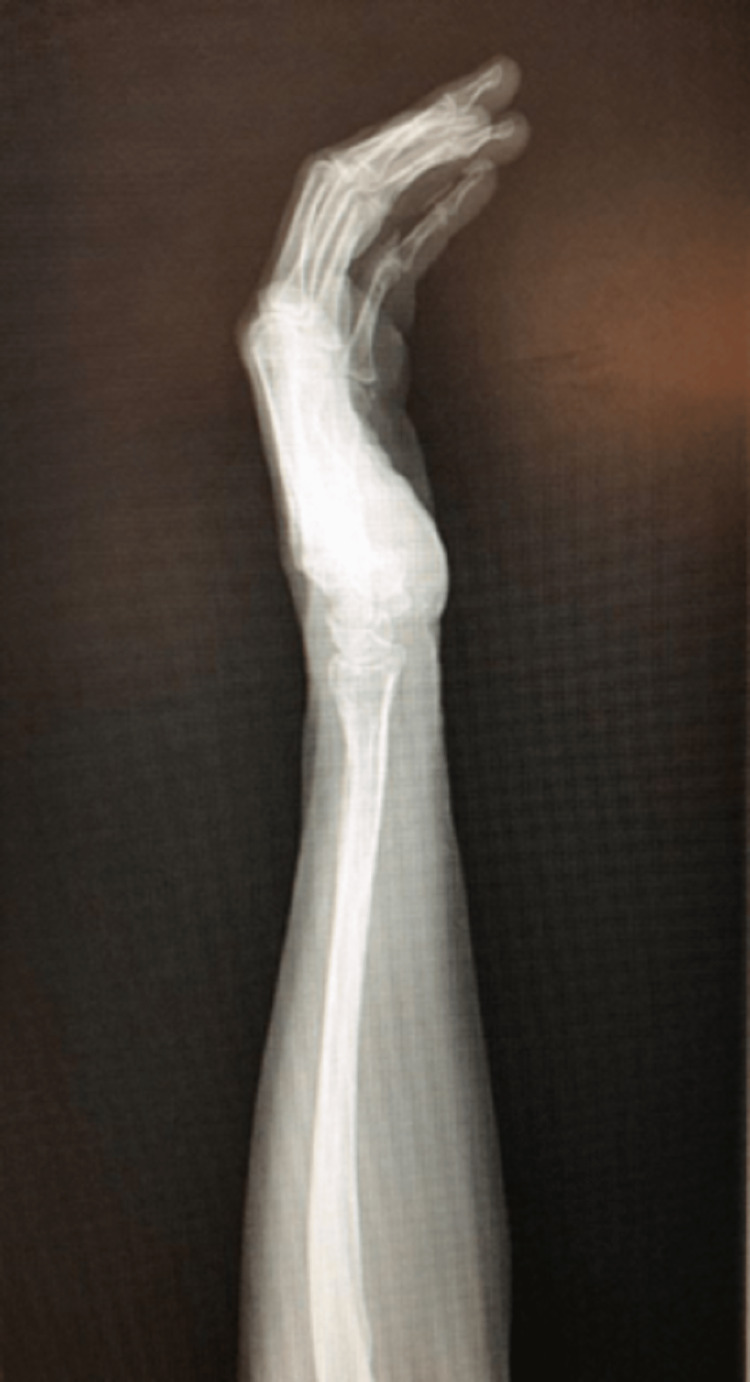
Left forearm and wrist radiograph - lateral view Radiograph of the patient’s left distal forearm and wrist in a lateral view. This radiograph does not show any immediate structural defects of the patient’s left radius, ulnar, carpal, and phalangeal bones.
